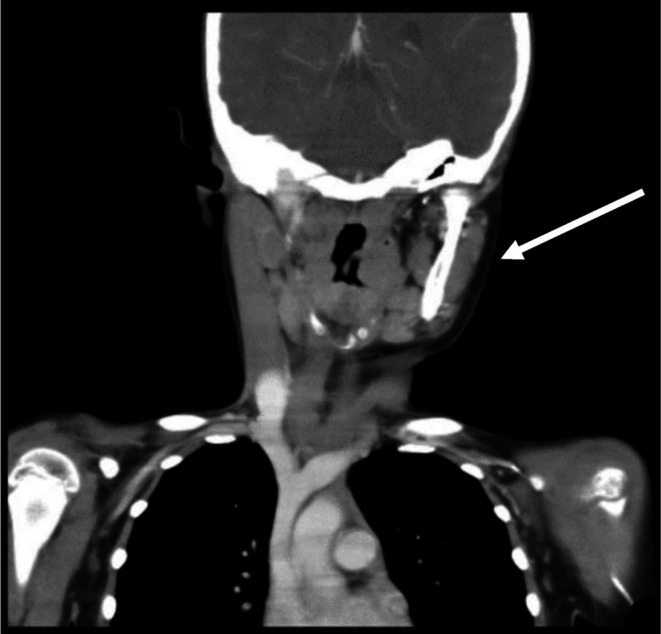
Coronal CT view demonstrating left tonsillar mass with associated ipsilateral cervical lymphadenopathy.
